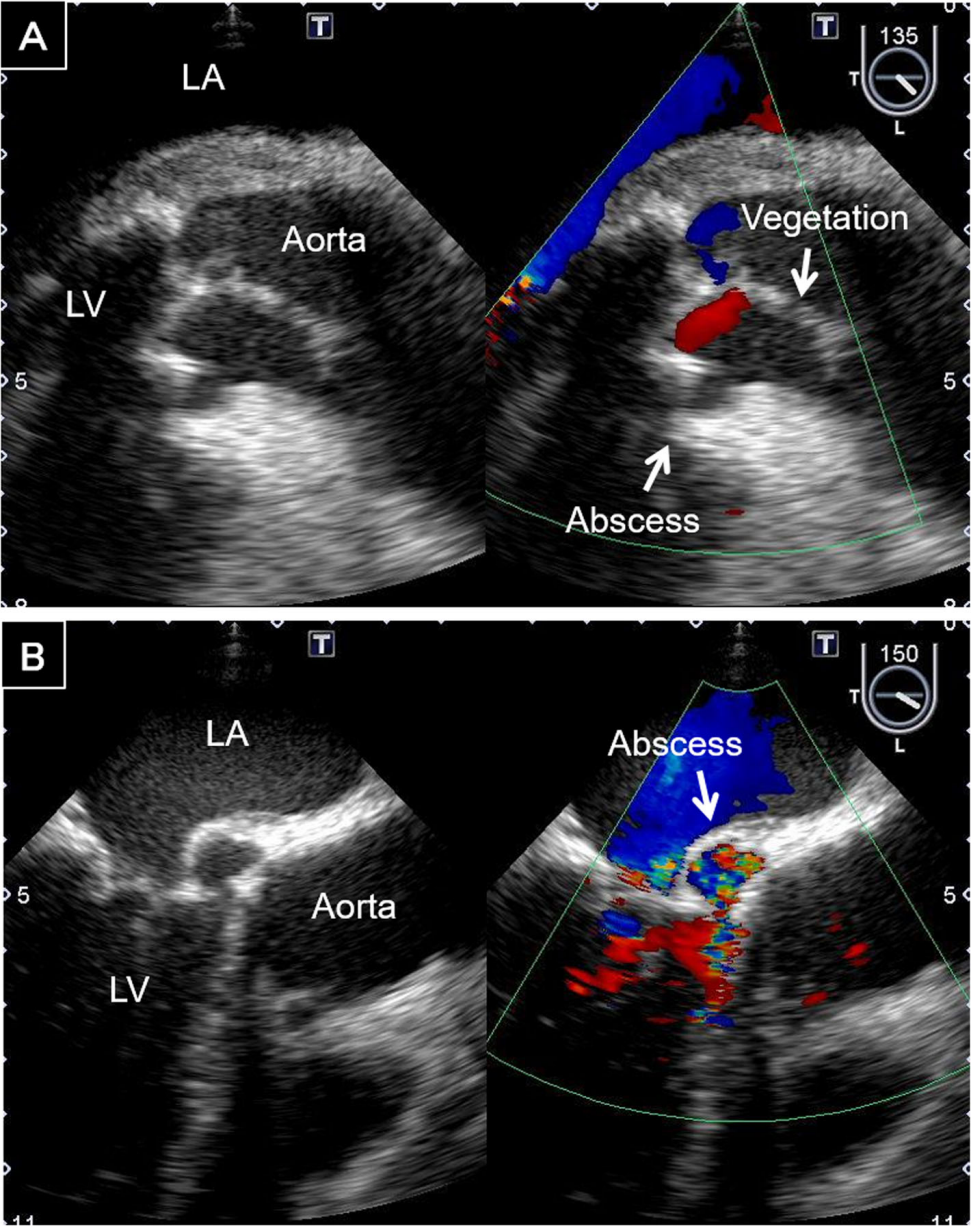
Transoesophageal echocardiogram. a A periannular abscess in the anterior annulus and vegetation growing from the aortic valve. b A periannular abscess in the posterior annulus. LA, left atrium; LV, left ventricle
